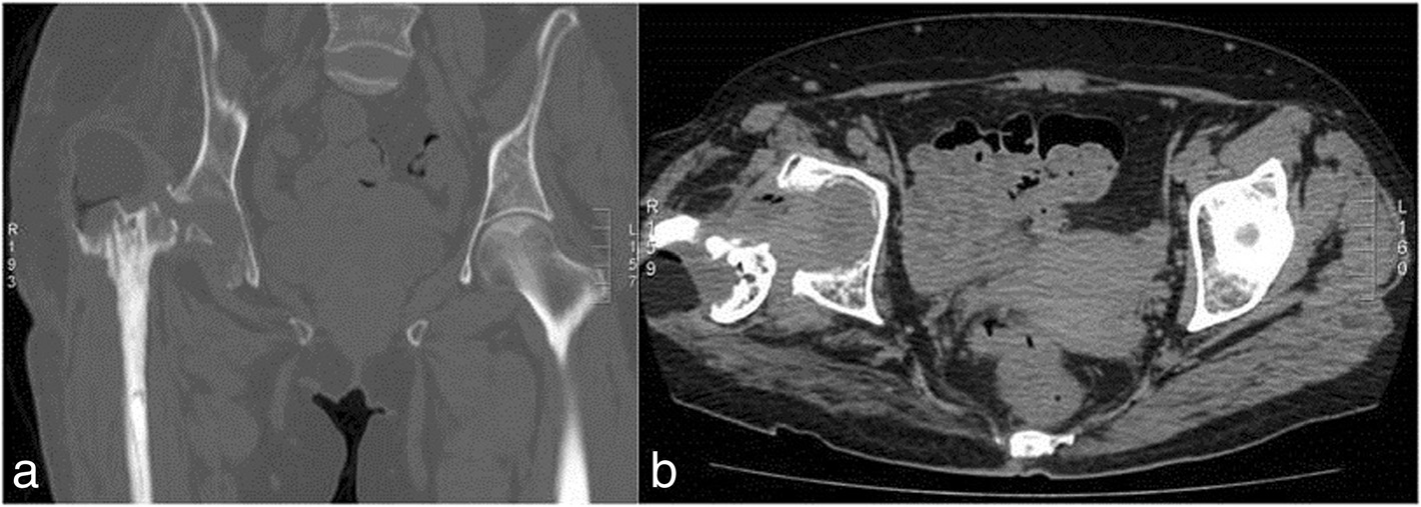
Computed tomography. a) Coronal and b) axial images showing fluid around the spacer, femoral inflammation, and spacer dislocation.
